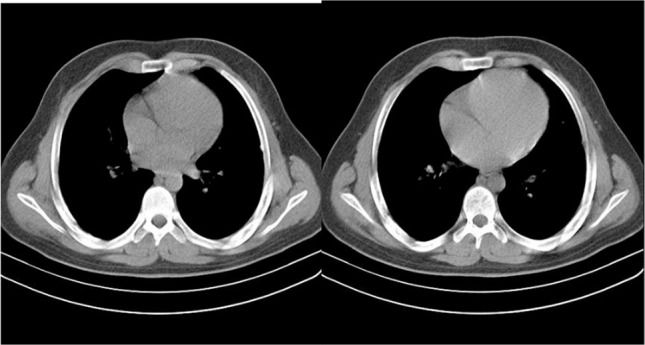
Chest CT one month (left) and three months (right) later
